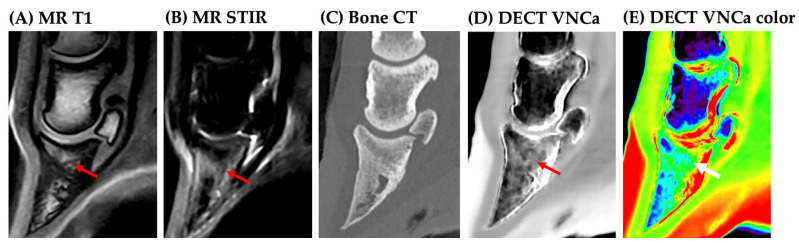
A 4-year-old Irish Cob with septic osteitis and a diffuse bone edema-like lesion (BME) of the distal phalanx (arrow) (post-mortem). (A) Diffuse T1-hypointensity of the distal phalanx; (B) diffuse short-tau recovery sequence hyperintensity of the distal phalanx; (C) standard bone CT—mildly increased bone density of the distal half of the distal phalanx; (D) DECT virtual-non-calcium map (VNCa) map—diffuse hyperdensity of the distal phalanx; (E) color-coded DECT VNCa map—BME is displayed in green, distinguishing it from normal fatty bone marrow, which appears blue.
